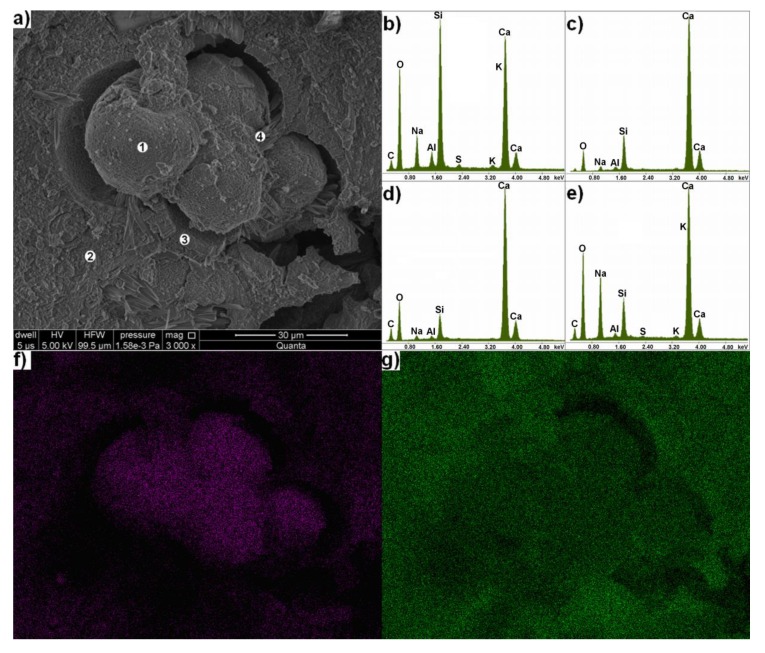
(a) a cracked grain of carbonate aggregate in mortar at 3000× magnification and X-ray microanalysis of elemental composition in point; (b) 1; (c) 2; (d) 3; (e) 4, and distribution maps of (f) Si; and (g) Ca.
